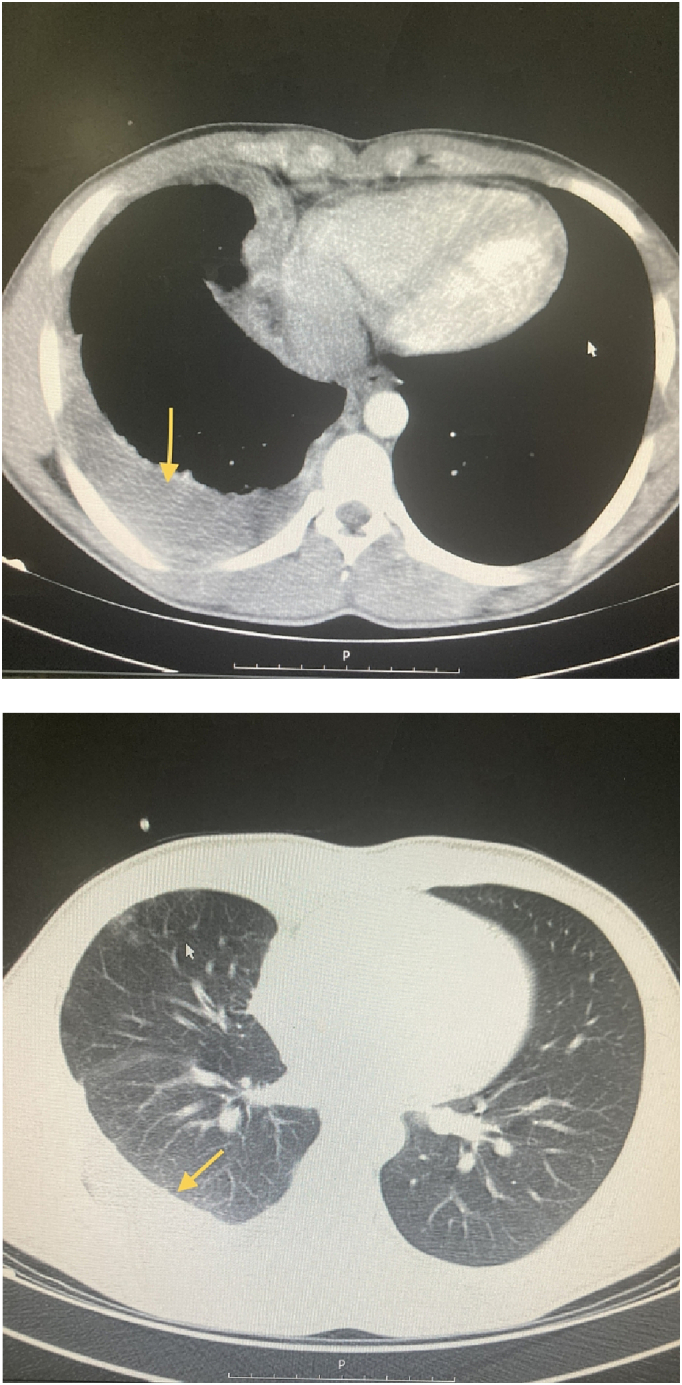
Chest CT just shows the plural effusion in right hemithorax.
